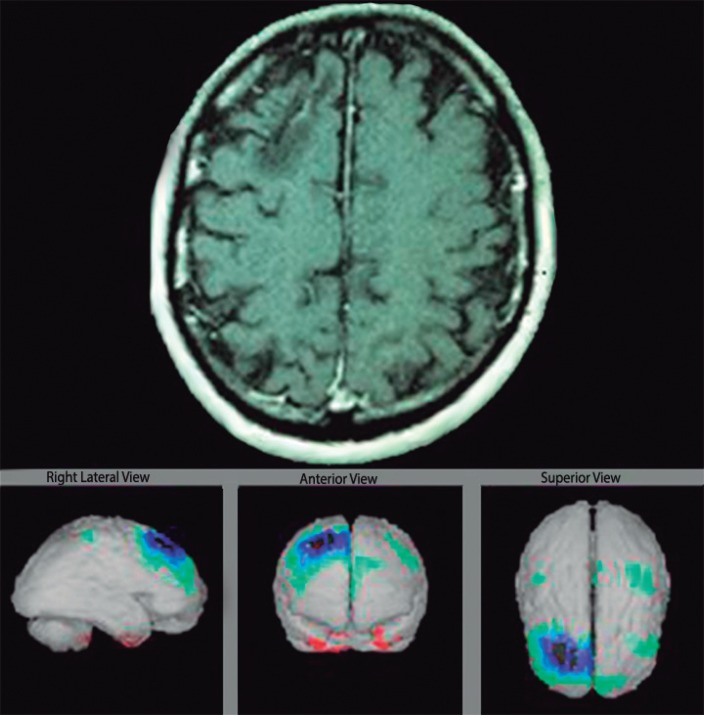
MRI and brain perfusion quantification of case 2.
